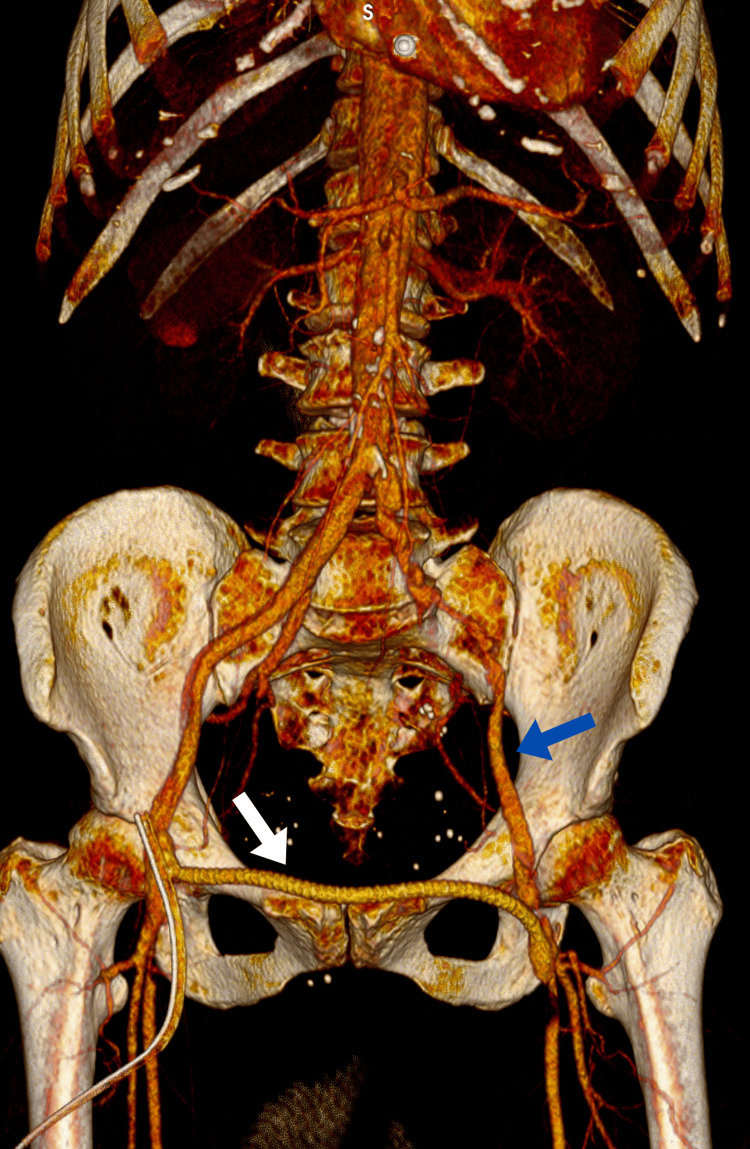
Postoperative 3D CT Angiographic Reconstruction The white arrow indicates a right-to-left femoro-femoral bypass graft. The blue arrow indicates restored perfusion in the left lower limb 3D, three-dimensional; CT, computed tomography
